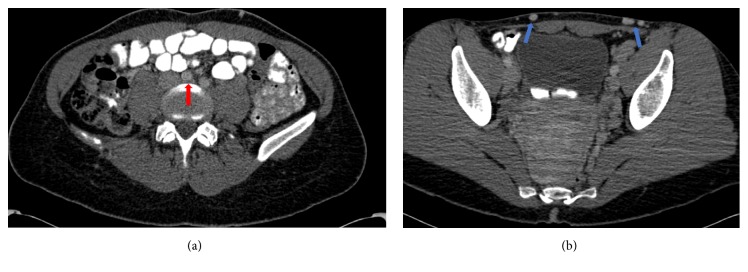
CT venogram of the abdomen pelvis revealed (a) compression of the left common iliac vein by the right common iliac artery (red arrow), and (b) abdominal and left groin varicosities (blue arrows).
